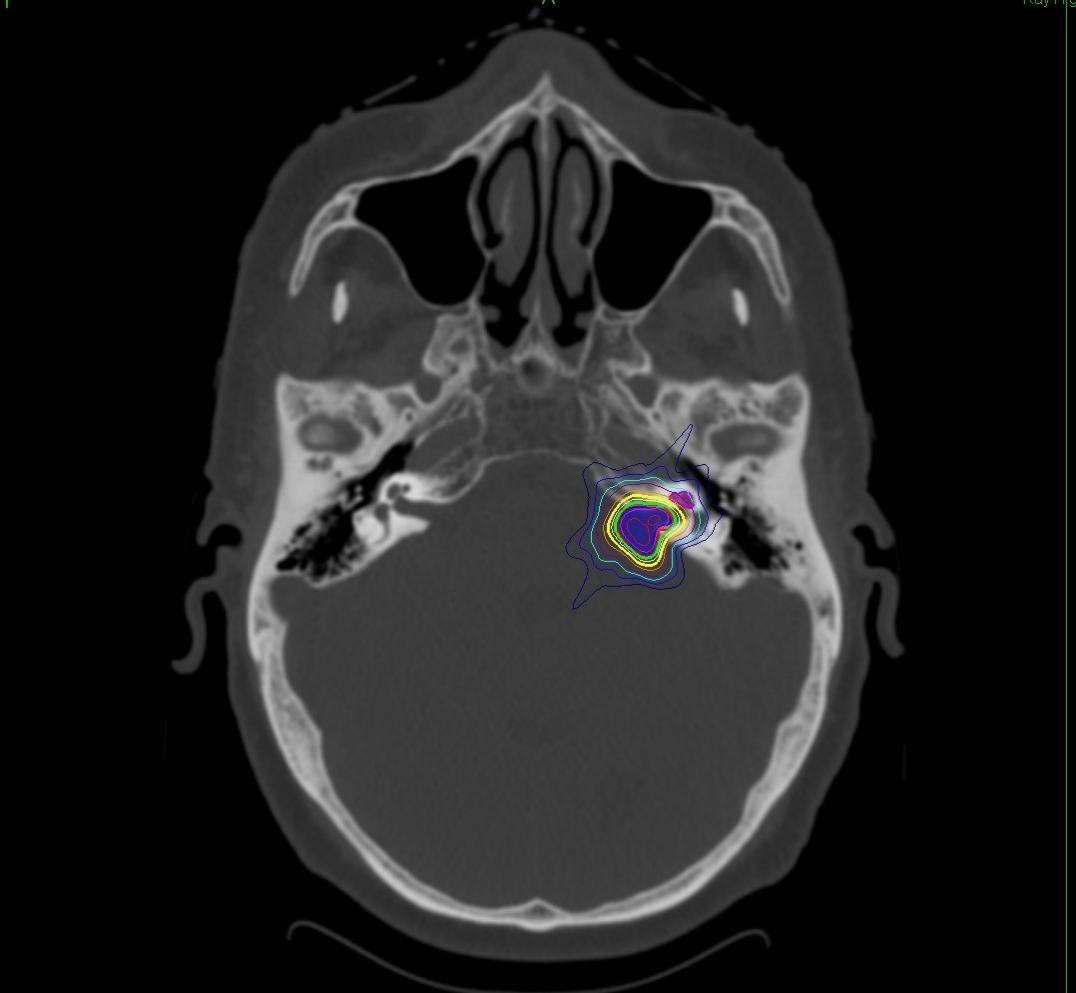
Treatment planning information from an example left-sided vestibular schwannoma patient. Axial computed tomography (CT) scan co-registered with magnetic resonance imaging (MRI) showing the treatment plan. The outlined tumor volume (blue shading) was expanded by 1.25 mm to create the planning target volume (PTV) (red shading). A high-degree of conformality was achieved between the PTV and the prescription isodose line (green contour line representing 2100 cGy). The surrounding isodose contours show sharp dose fall-off away from the tumor target with good sparing of the cochlea (magenta shading).
